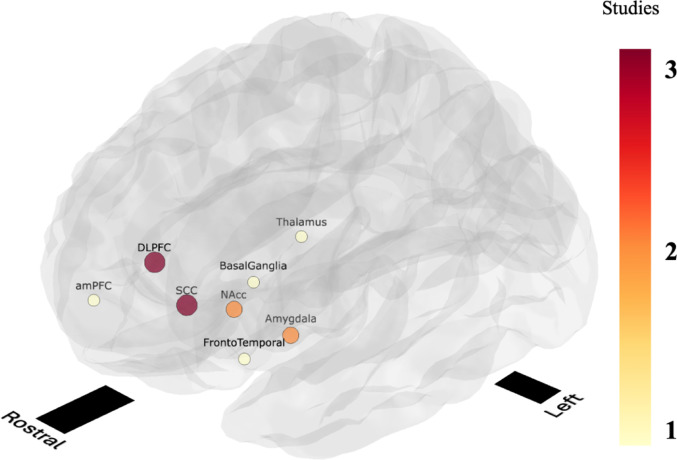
Neural targets of selected focused ultrasound neuromodulation studies for psychiatric indications
